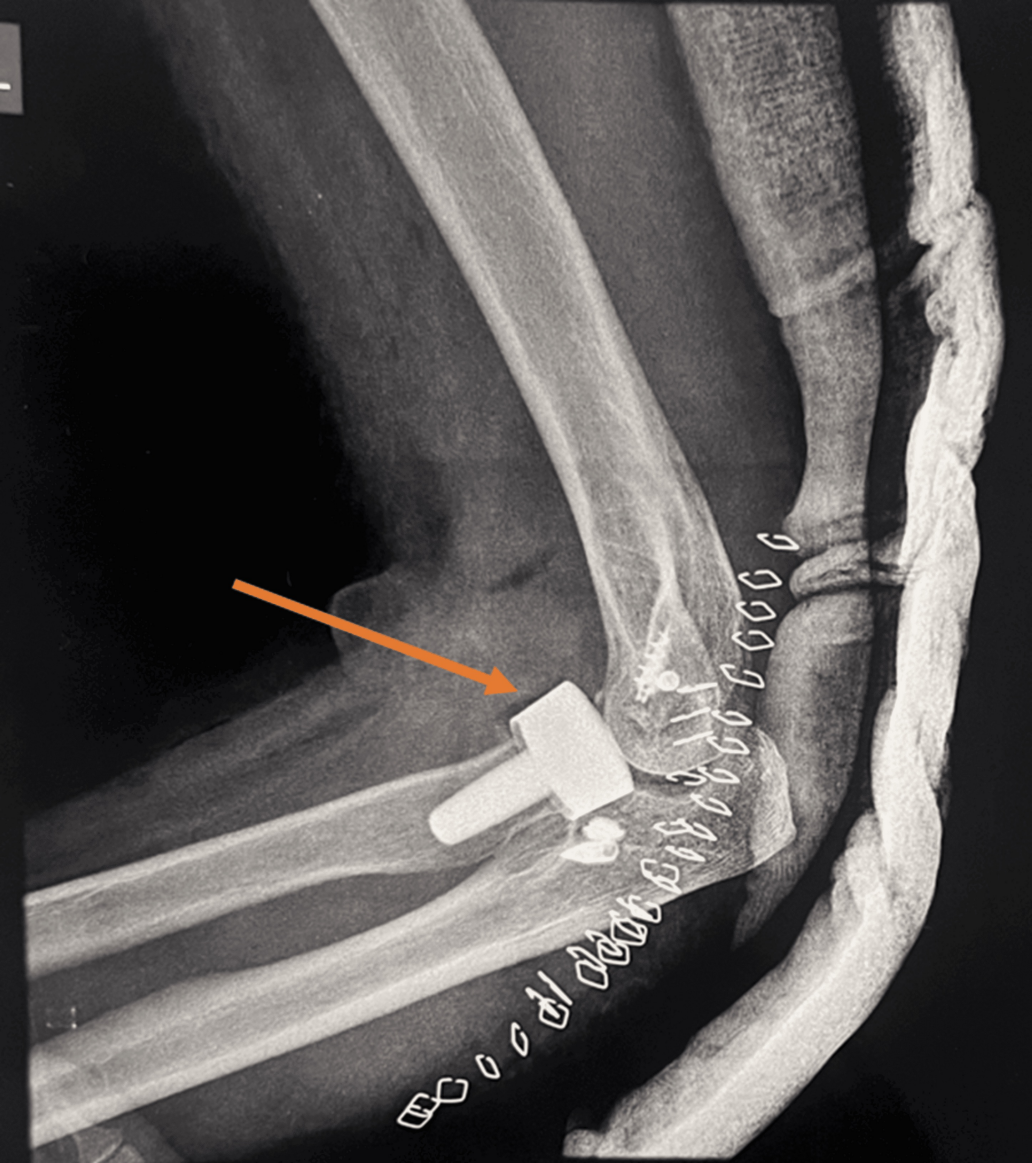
Postoperative radiograph showing Mason type IV radial head fracture treated with a radial head prosthesis
